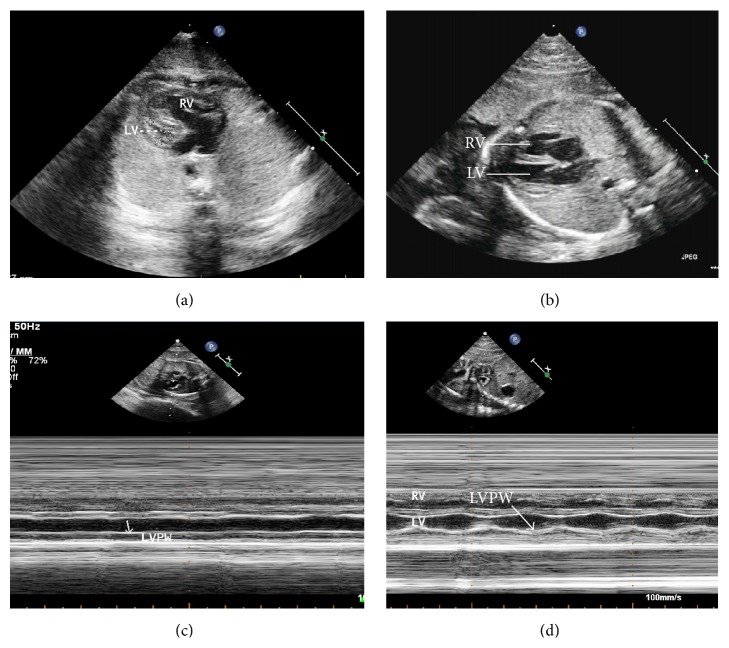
HLHS fetal echocardiogram images at 33 weeks of gestation. Fetal echocardiogram in a 4-chamber view, demonstrating that both the end-diastolic length and the diameter of left ventricle (LV) decreased (a) as compared with the normal heart in same gestational age (b), while the right ventricle (RV) diameter increased (a), resulting in a notably higher diameter ratio of the RV to LV. M mode echocardiogram image showed the poor contractility of LV (c, white arrow) as compared with the normal heart (d, white arrow). LV: left ventricle; RV: right ventricle; and LVPW: left ventricular posterior wall.
